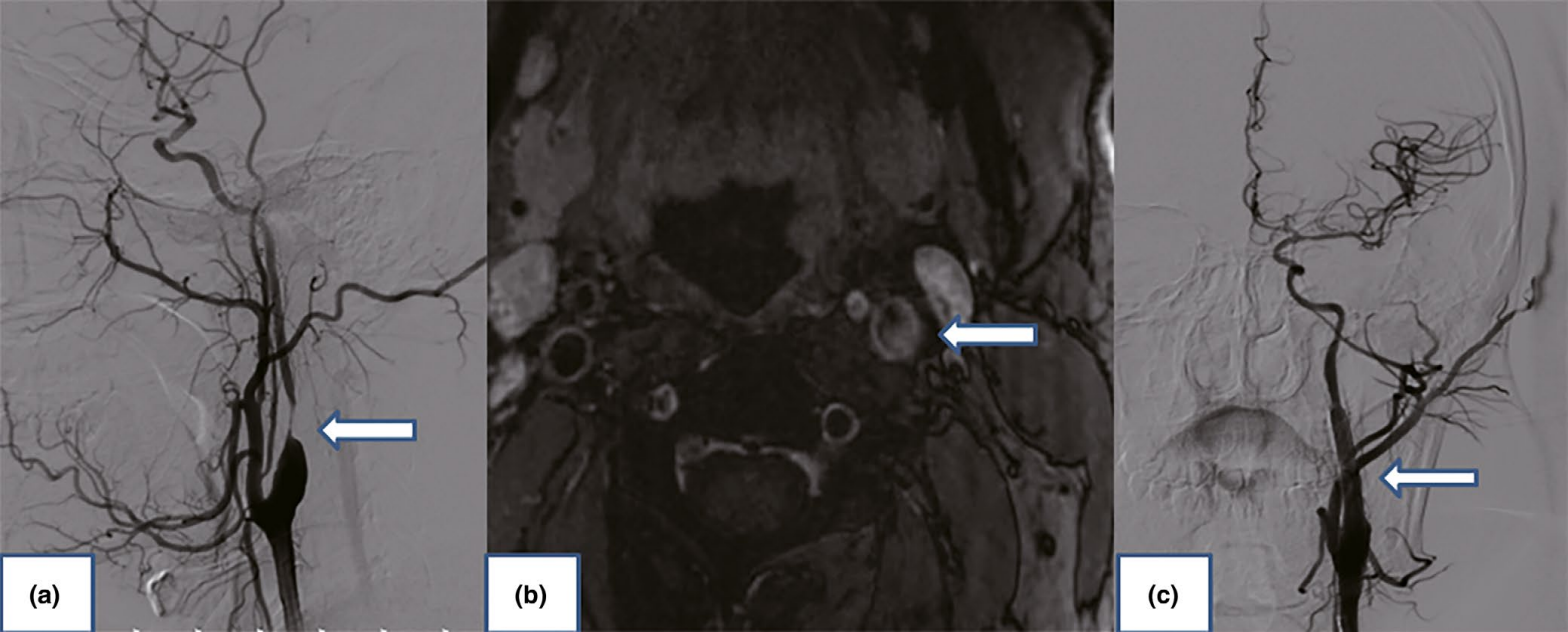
(a) Digital subtraction angiography showing the significant stenosis of the left internal carotid artery (arrow). (b) High‐resolution magnetic resonance imaging showed ruptured fibrous caps, large lipid core, intraplaque hemorrhage, and ulcer (arrow). (c) Completion angiogram after angioplasty and stenting of the left internal carotid artery showing the excellent restoration of flow (arrow)
